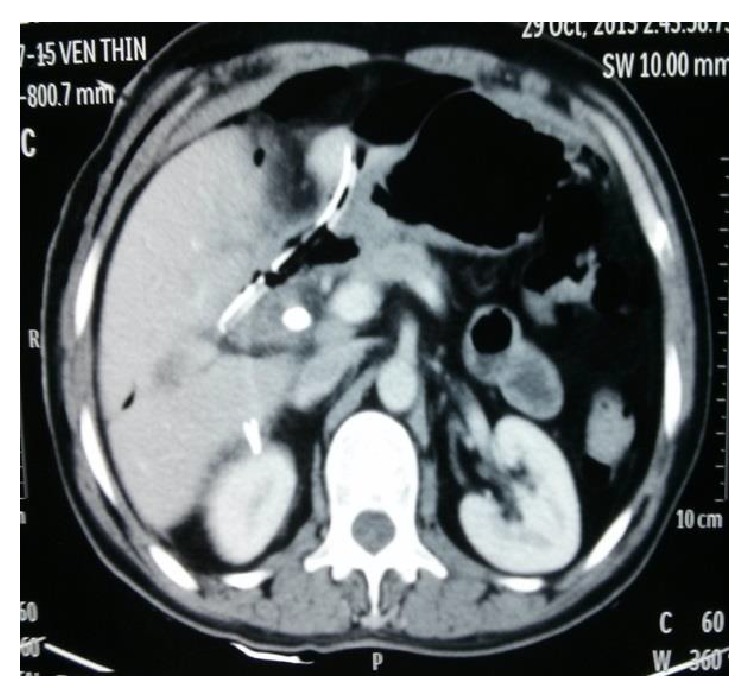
CT scan showing the drain in the 2nd part of duodenum.
